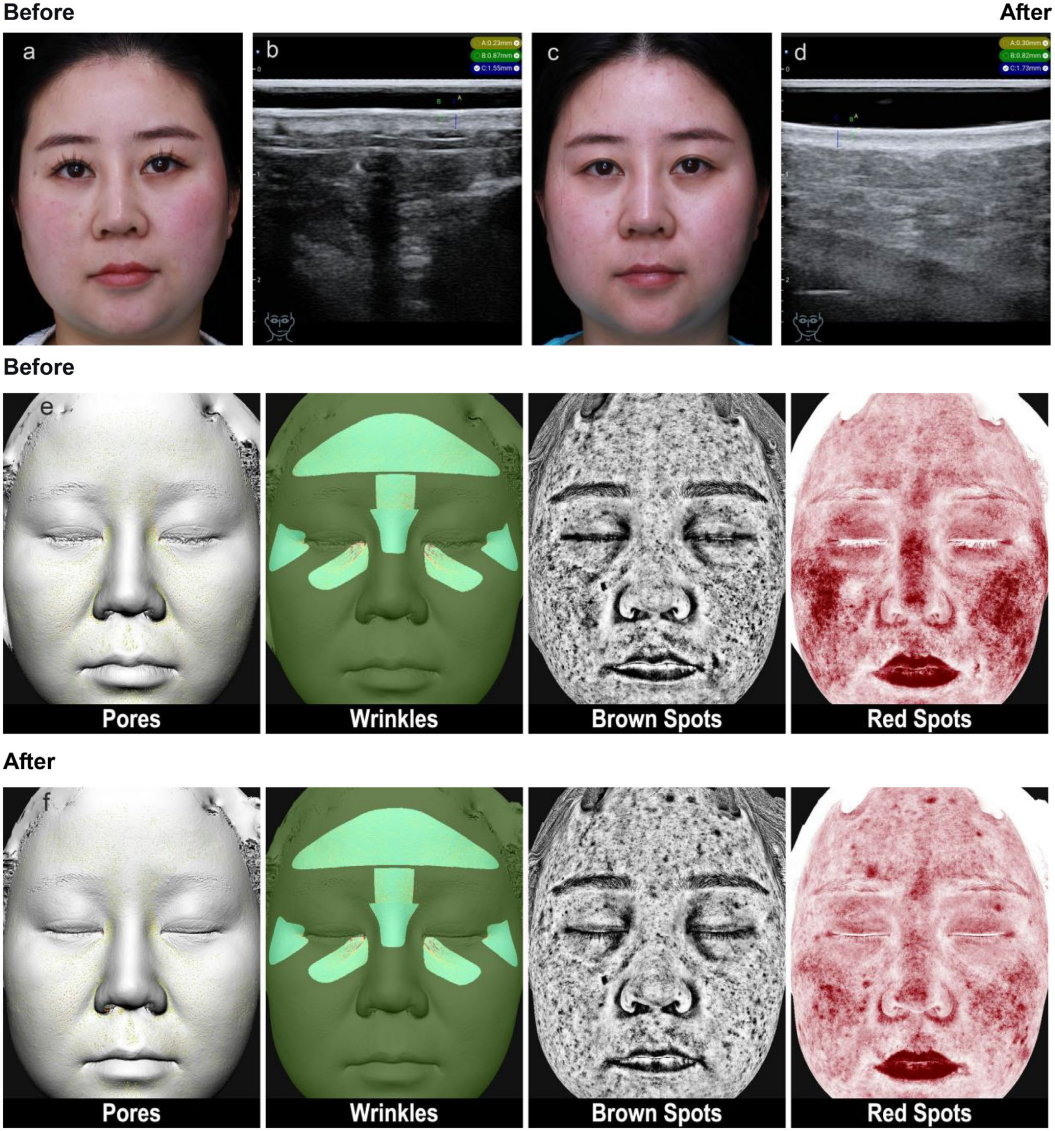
Typical case 2. “33‐year‐old female, facial sensitive skin, redness, thin stratum corneum, mild wrinkles around the eyes.” (a) “Clinical image before PRP treatment.” (b) “High‐frequency ultrasound (HFUS) measurement of epidermis, SLEB, and dermal thickness in the perioral area.” (c) “Clinical image four weeks after the third PRP treatment.” (d) “HFUS measurement of skin thickness in the perioral area four weeks after the third PRP treatment.” (e, f) “Skin analyzer images of Pores, Wrinkles, Brown Spots, Red Spots before and four weeks after the third PRP treatment.”
